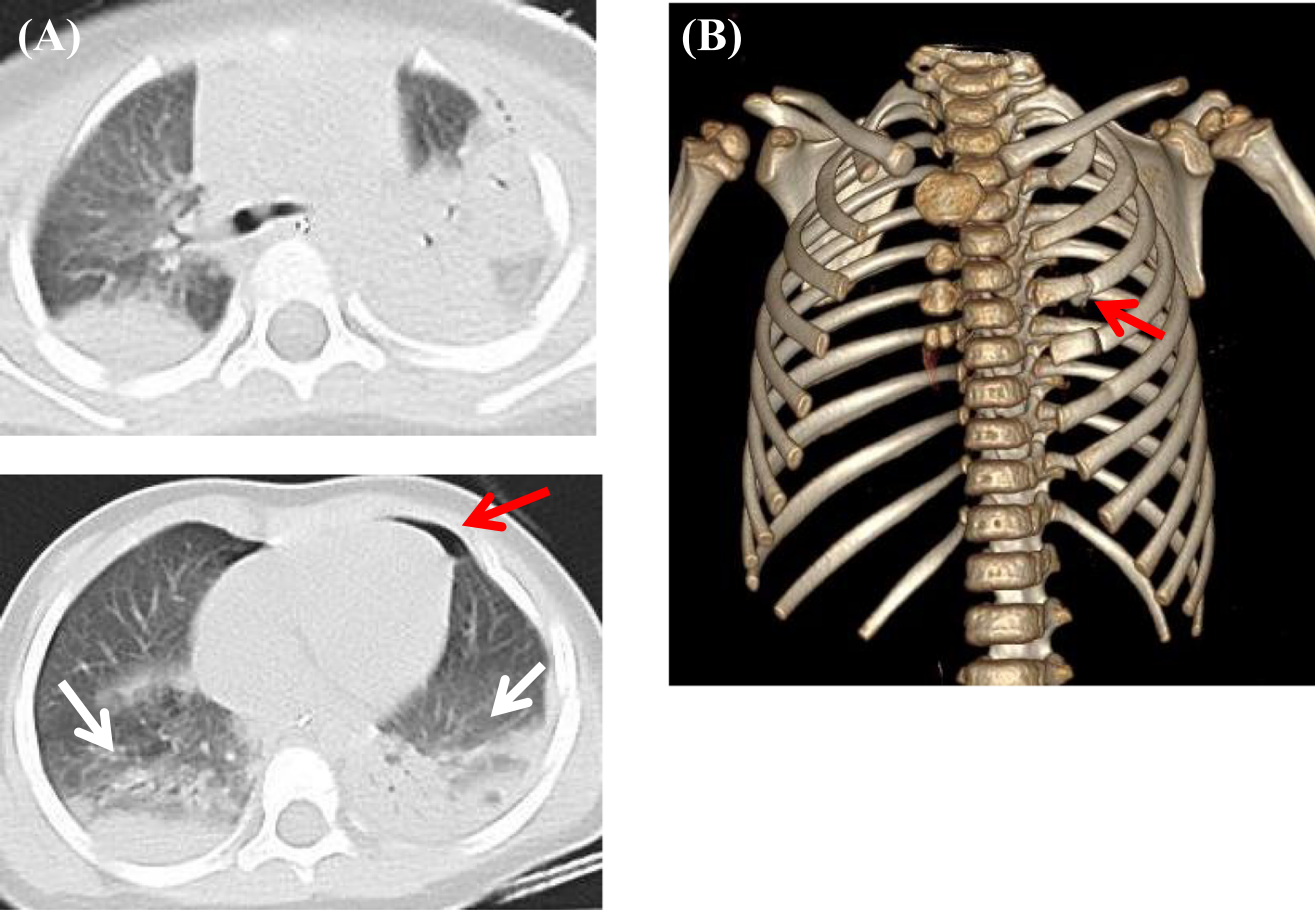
Chest CT of Case 2 on Admission. a Two-dimensional axial CT scan showed lung contusions (*white arrows*) and left hemopneumothorax (*red arrows*). b Three-dimensional reconstruction of the CT scan showed fractures of the third to fifth ribs on the left (*red arrows*)
